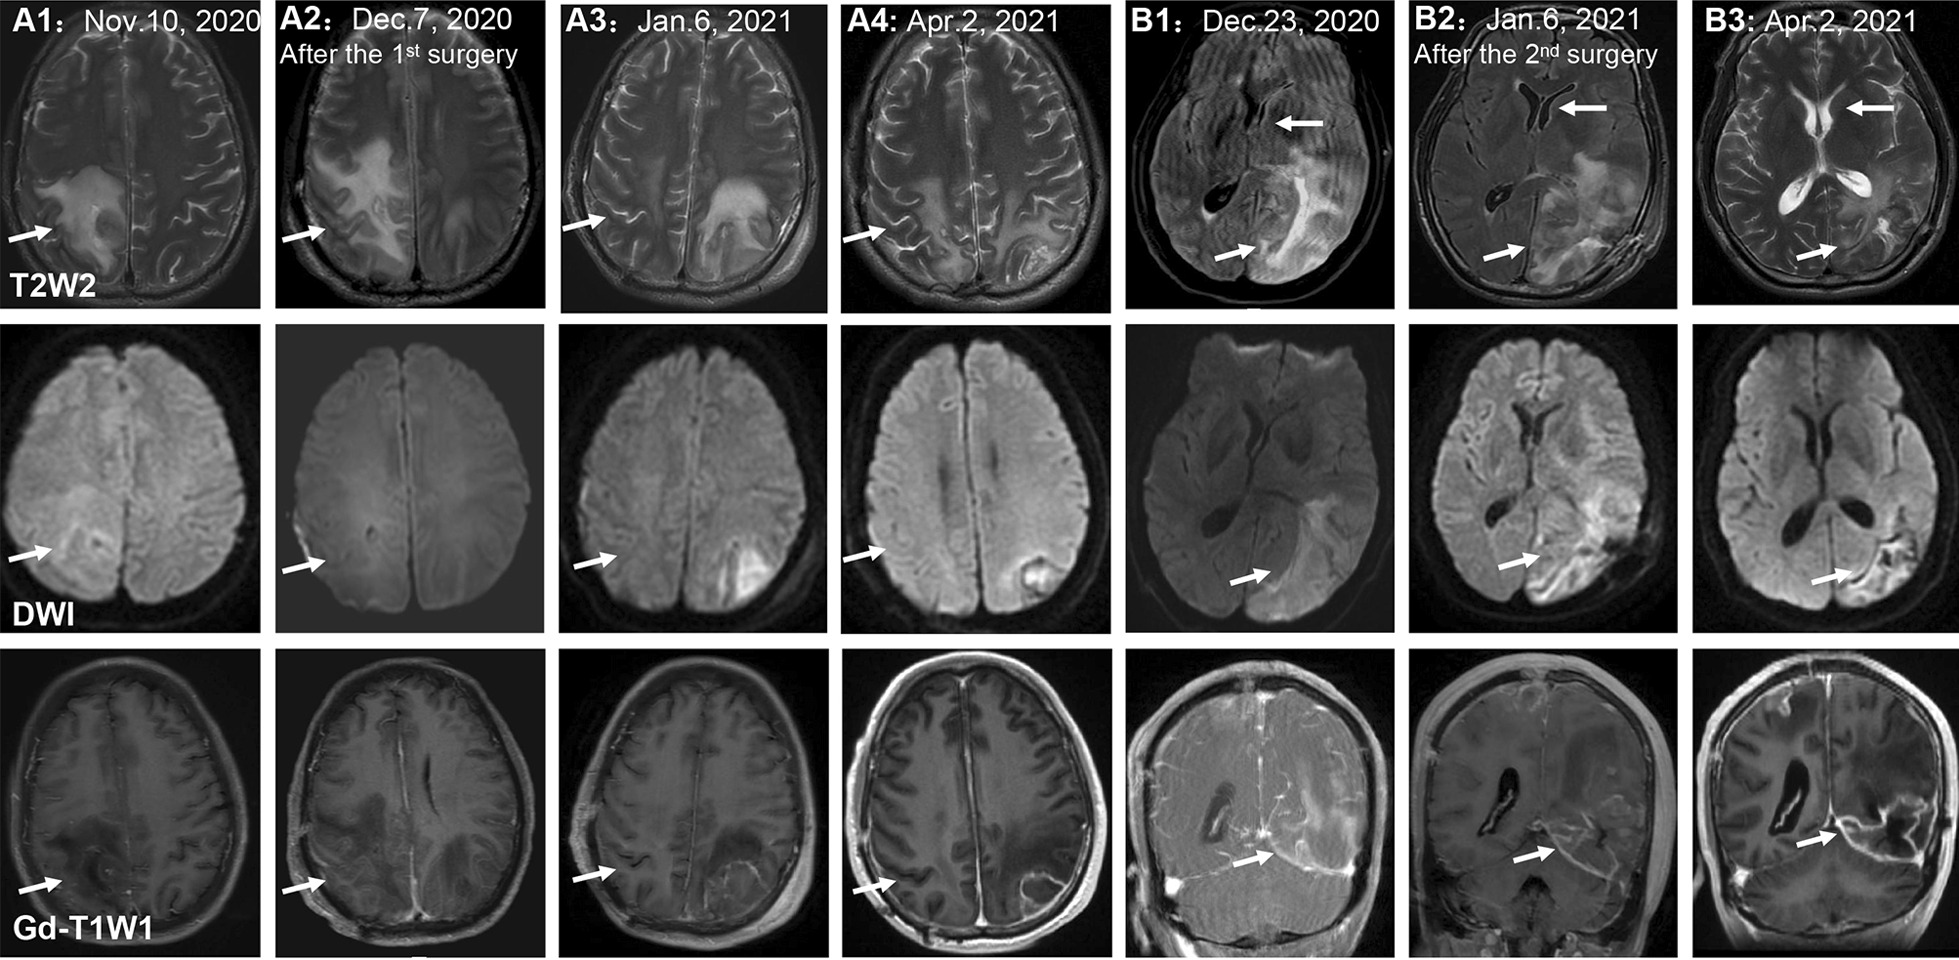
The dynamic changes of brain magnetic resonance imaging (MRI). A1–A4 The lesions of the right parietal lobe were obviously getting smaller on T2-weighted image (T2W2). B1–B3 The lesions of the left occipital lobe and the left cerebral subfalcine herniation became better slightly. Both lesions of the right parietal lobe and the left occipital lobe showed high signal intensity on T2W2, obvious high signal intensity on diffusion-weighted image (DWI) and enhancement on gadolinium-enhanced T1-weighted image (Gd-T1WI) in the left occipital lobe lesions, milder enhancement in the right parietal lobe
